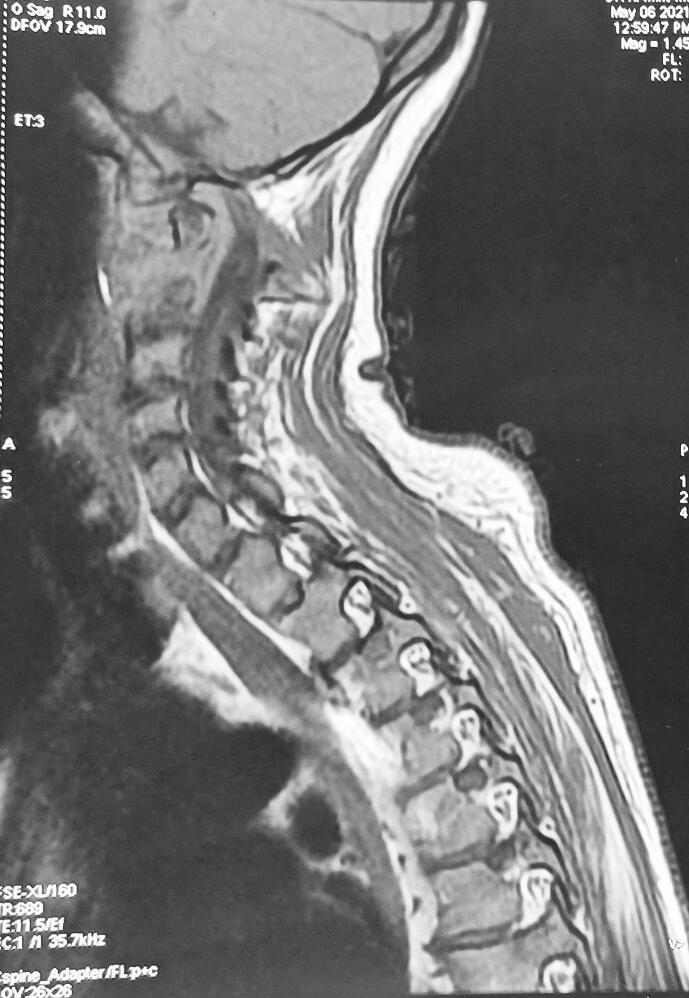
Sagittal T2-weighted MRI of the cervical spine showing marked atlantoaxial instability with anterior displacement of the atlas (C1) over the axis (C2). There is significant narrowing of the spinal canal at the craniovertebral junction, resulting in spinal cord compression. The spinal cord appears deformed at this level, and a hyperintense signal change within the cord suggests edema or early myelomalacia. Degenerative changes and normal alignment are noted in the lower cervical spine.
